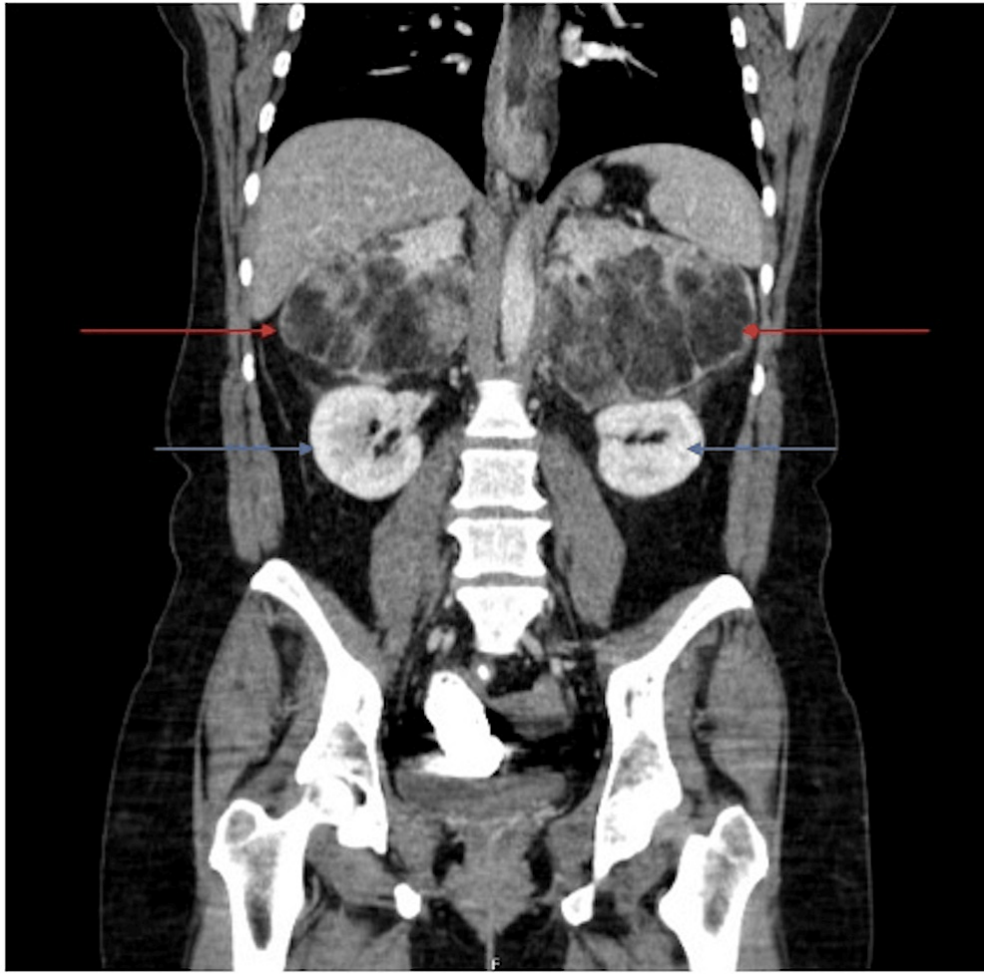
Anterior view of CT of abdomen and pelvis with contrast. Bilateral adrenal myelolipomas (red arrows) and inferiorly deviated kidneys are visible (blue arrows). CT: computed tomography
